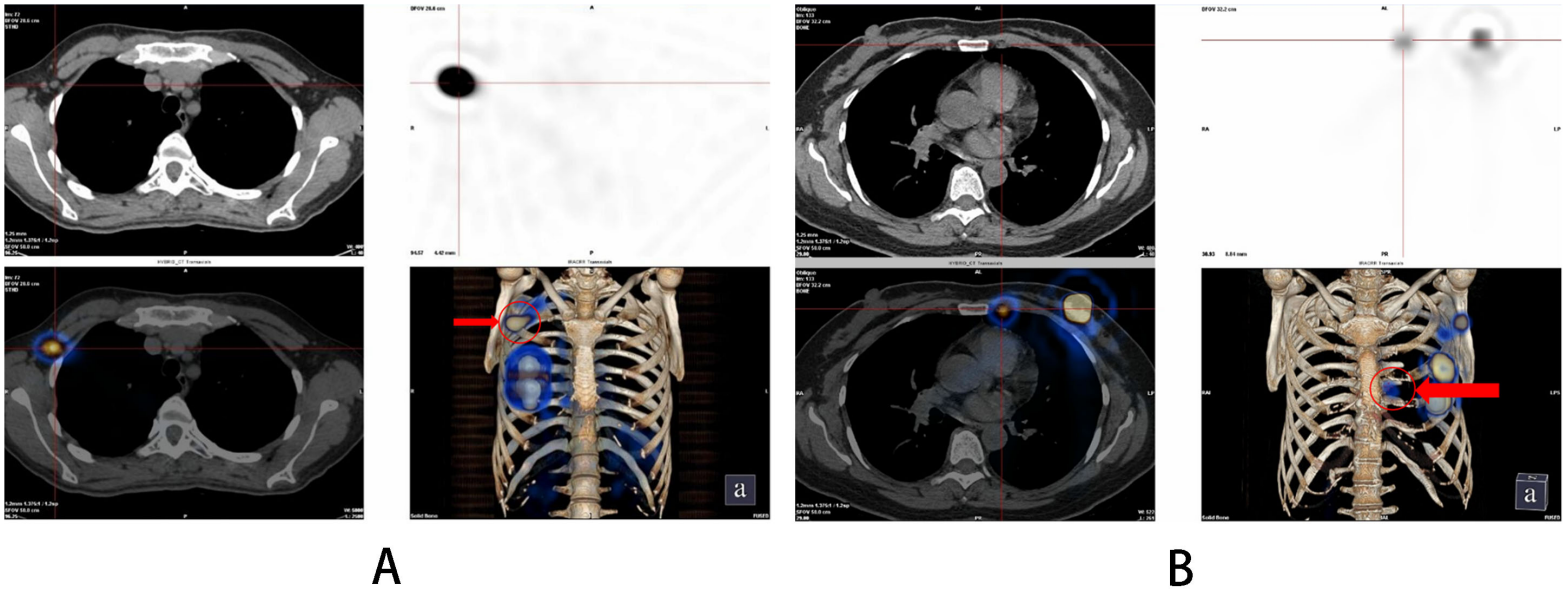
SPECT/CT imaging results after 1-3 hours after injection of 99mTc-rituximab. (A) Sentinel lymph nodes; (B) Internal mammary lymph nodes.
